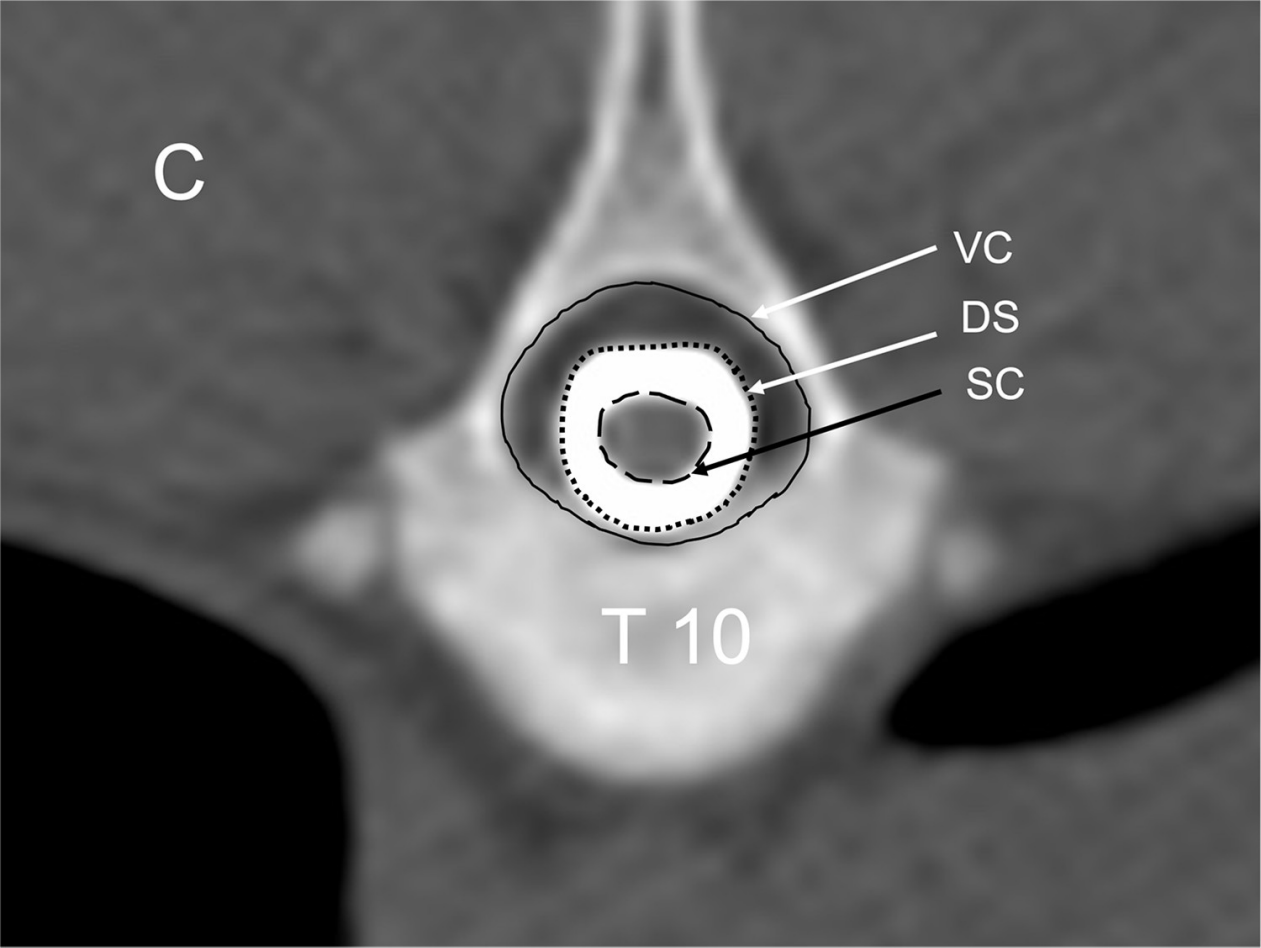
Transverse CT myelogram image showing manual delineation for area measurements. Spinal cord (SC), dural space (DS), and vertebral canal (VC). This site has the qualitative designation of C for continuous contrast filling of the subarachnoid space. C = continuous and concentric filling of the subarachnoid space.
